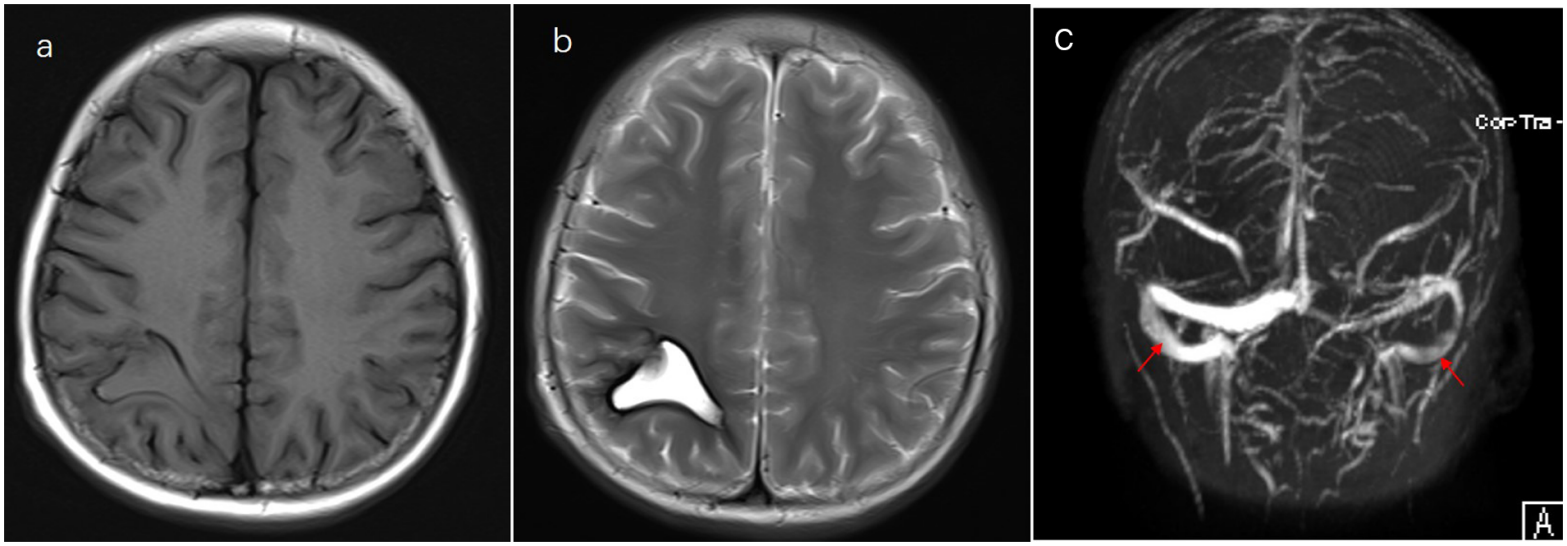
Evolution of changes on follow-up. (a) T1- and (b) T2-weighted MRI and (c) magnetic resonance venography imaging performed after the treatment.
